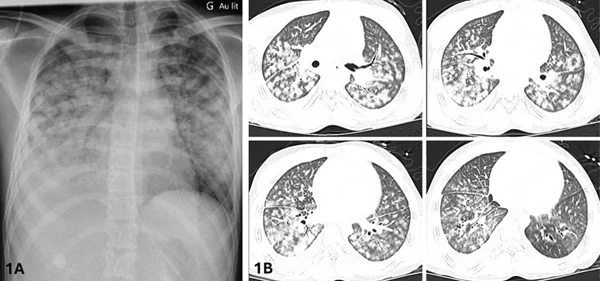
(A) Chest x‐ray on PICU admission showed diffuse bilateral air‐space opacities with bronchogram and right pleural effusion. (B) Thoracic CT scan showed bilateral and symmetrical diffuse alveolar opacities suggestive of alveolar hemorrhage and areas of ground glass suggestive of underlying infection and pleural effusions.
